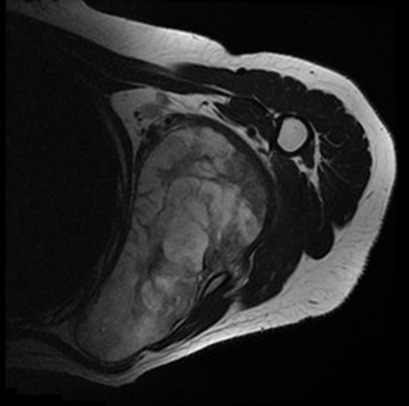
MRI of the shoulder lesional process of the sub-scapûlar fossa in hypersegnal T2 heterogenepus containing multible incompleten partitions enhanced after injection of the gadolinium leading necroses beacher, this process is limited by a pseudo-capsule: axial section T2
